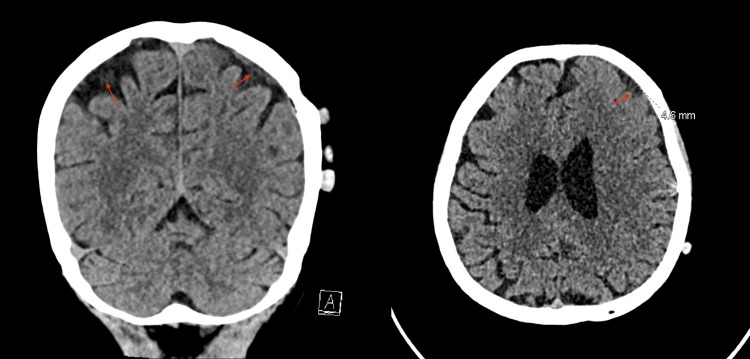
Eight weeks post-MMA embolization. Axial and coronal brain CT scans showing significant interval resolution of the subdural hemorrhage. MMA: Middle meningeal artery.
